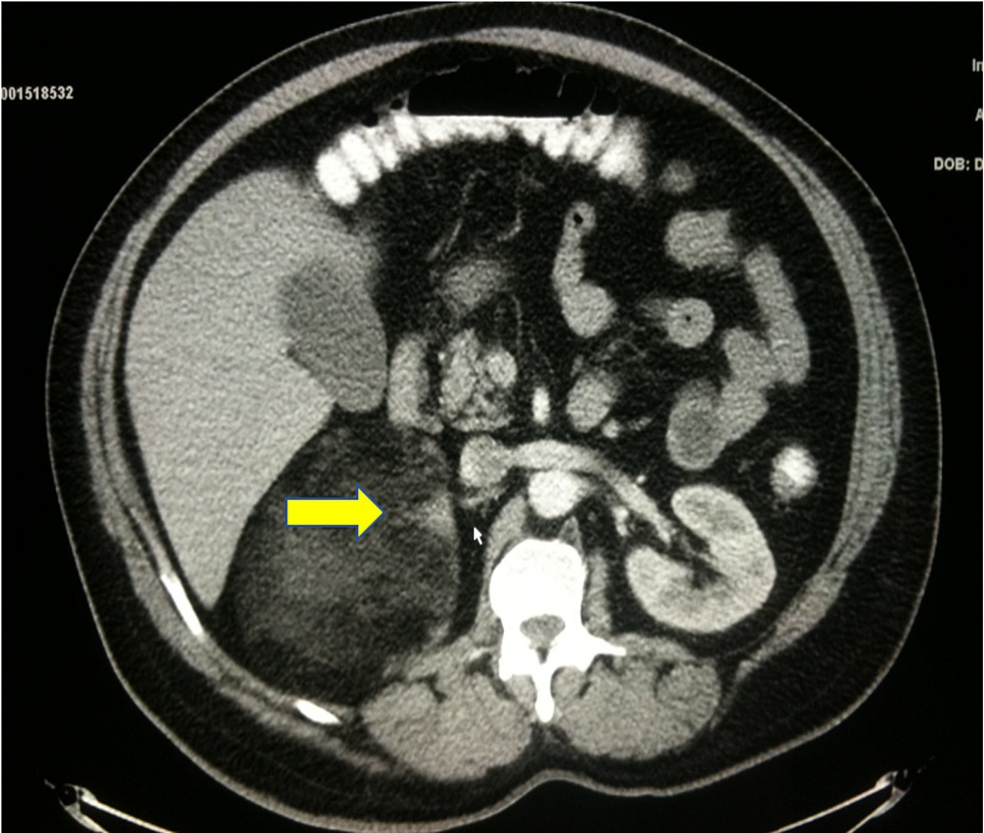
Contrast Enhanced CT Arterial Phase - Axial View Image demonstrates a large suprarenal, predominantly fatty mass with areas of soft tissue attenuation and focal hemorrhage (yellow arrow).
